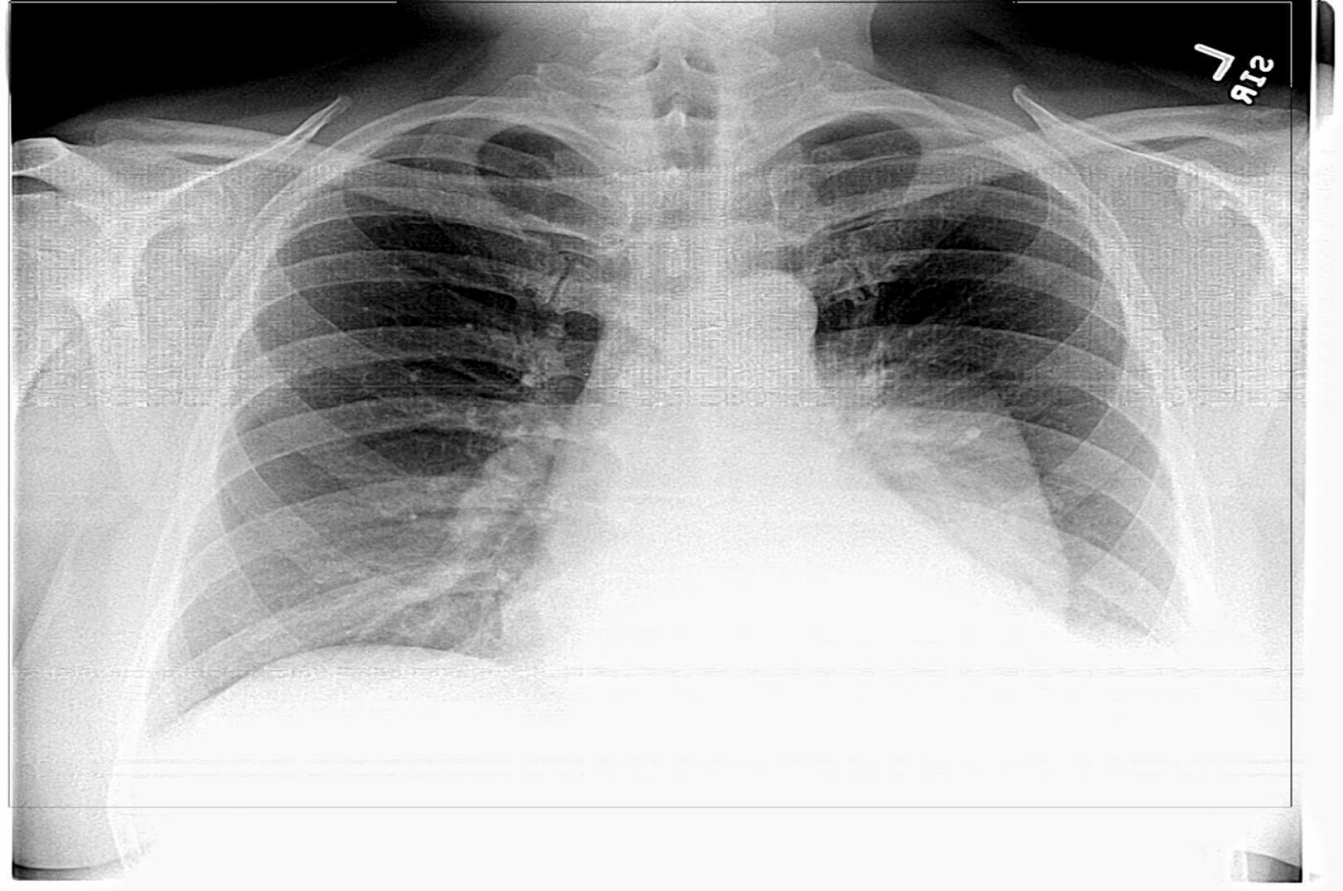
Chest radiograph with left-sided mass Chest radiograph in PA view showing a left-sided mass and pleural effusion. The mass is well-marginated and rounded without a meniscus.
